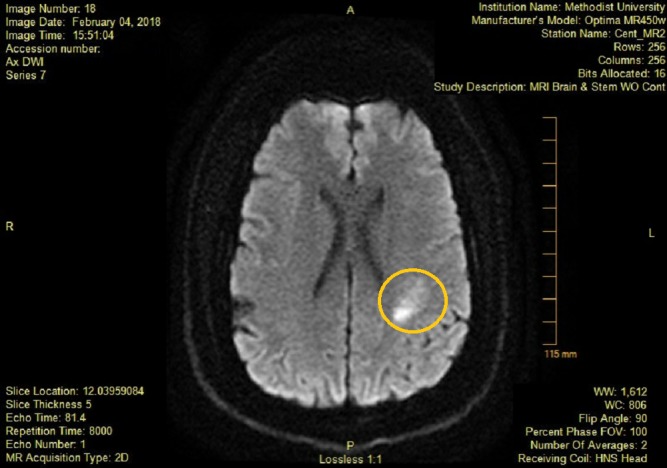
DW-MRI obtained after stroke symptoms showing new area of enhancement. Area of new stroke marked by circle. DW-MRI: Diffusion-weighted magnetic resonance imaging.
